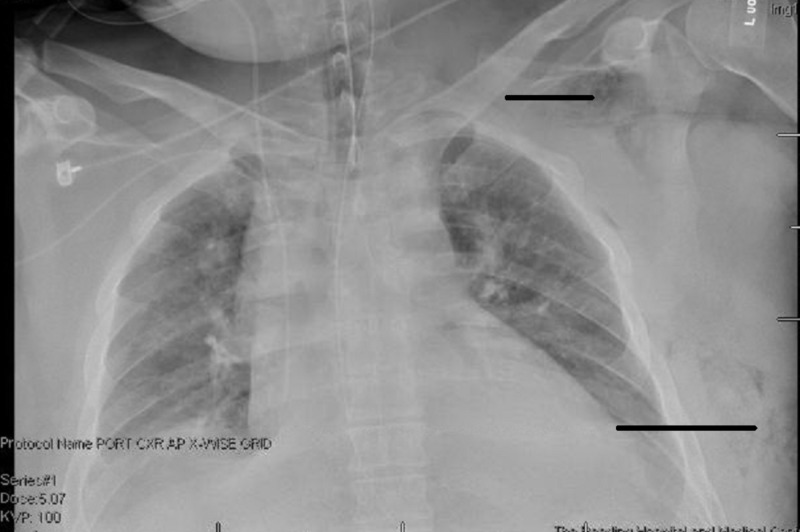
X-ray that shows soft tissue gas in the left chest
